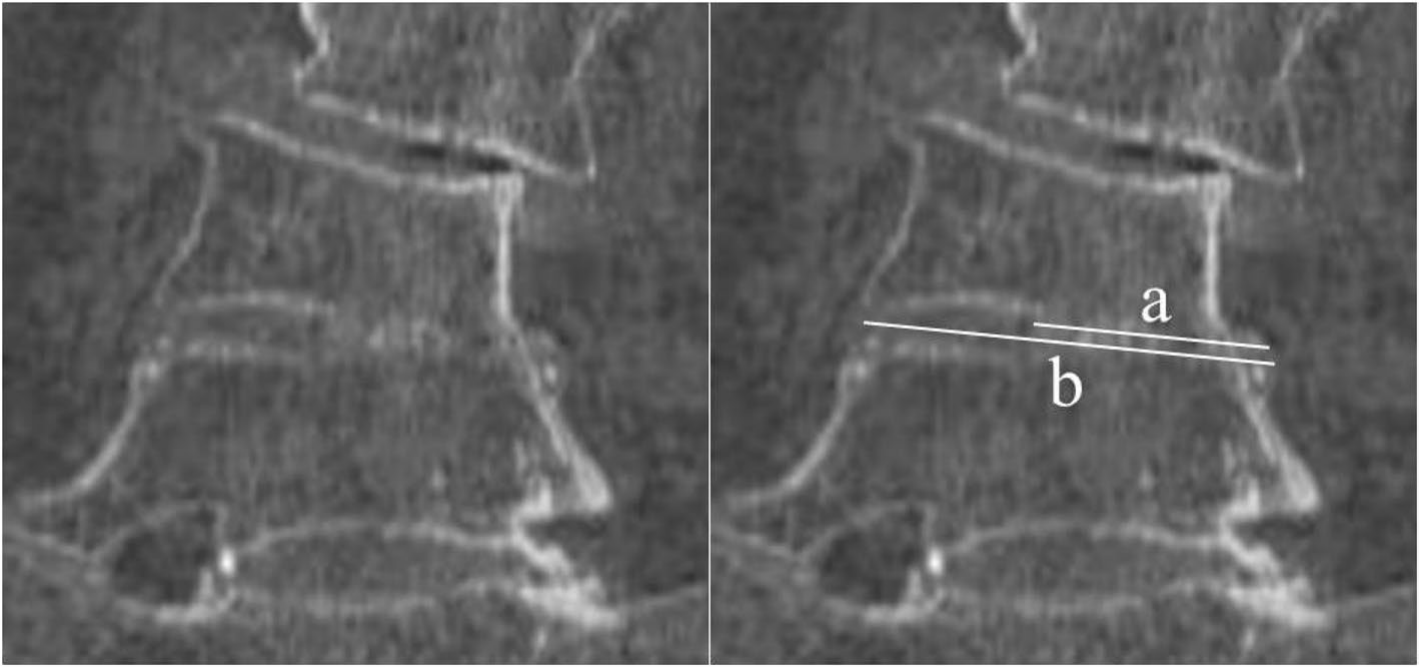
The fusion ratio is preoperatively calculated by dividing the length of the ossified portion of the intervertebral space (a) by the total length of the intervertebral space (b) on the exact coronal plane image using multiplanar reconstruction of computed tomography
